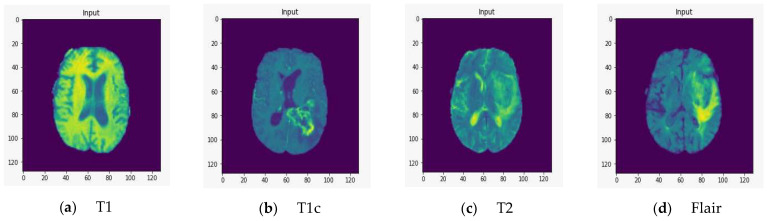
T1, T1c, T2, and FLAIR rendered at a resolution of 128 × 128 × 128.
